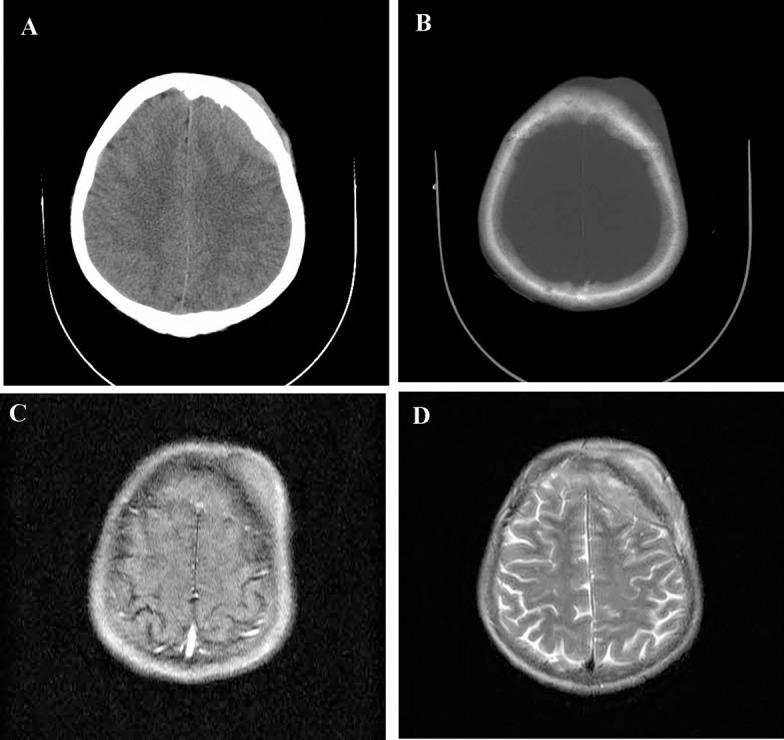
Axial CT scan (A,B), a calvarial lesion with both extra- and intracranial isodense soft tissue components with mild calvarial hyperostotic changes. T1-weighted (C) and T2 weighted (D) MR images show the lesion nearly isointense to gray matter.
